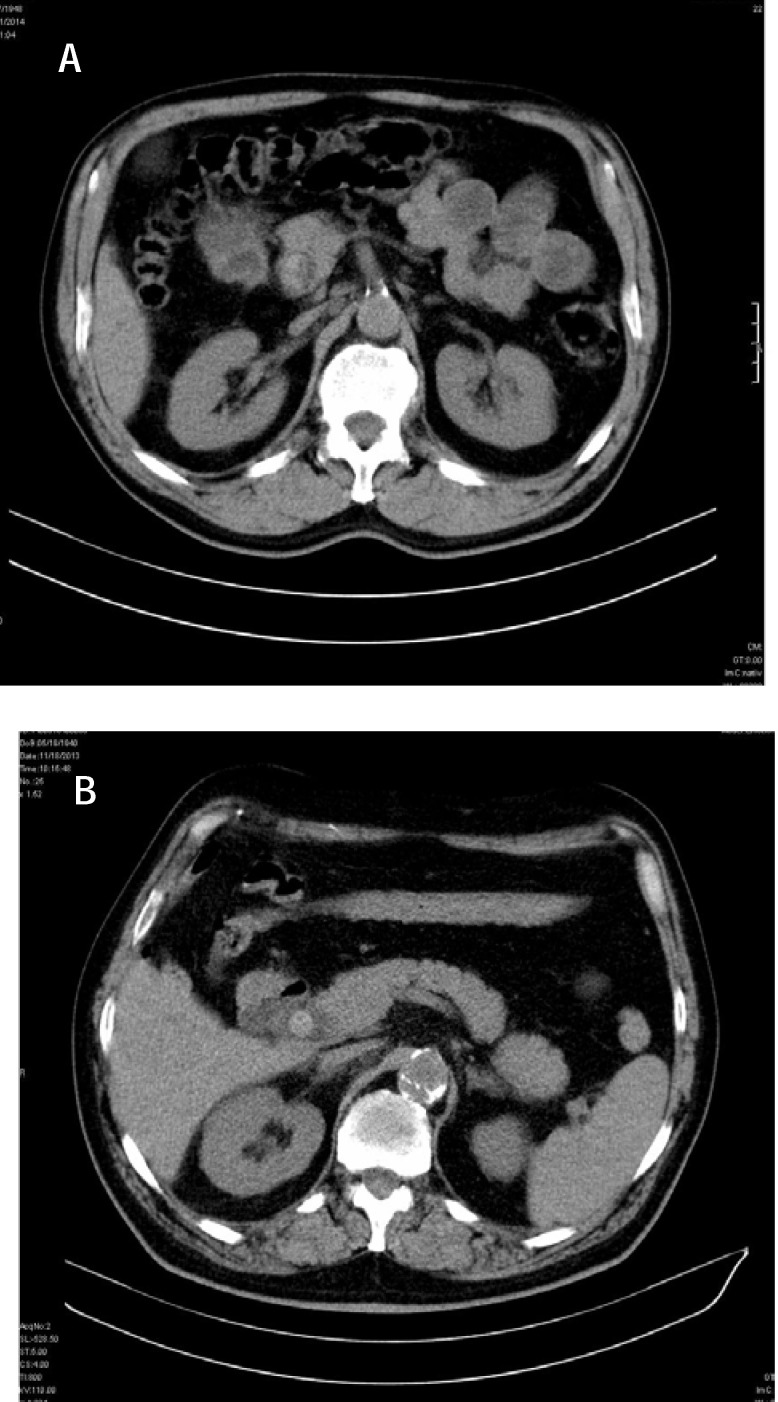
CT unenhanced: hyper attenuation calculi with dilated CBD (a) Terminal CBD; (b) prepancreatic CBD
